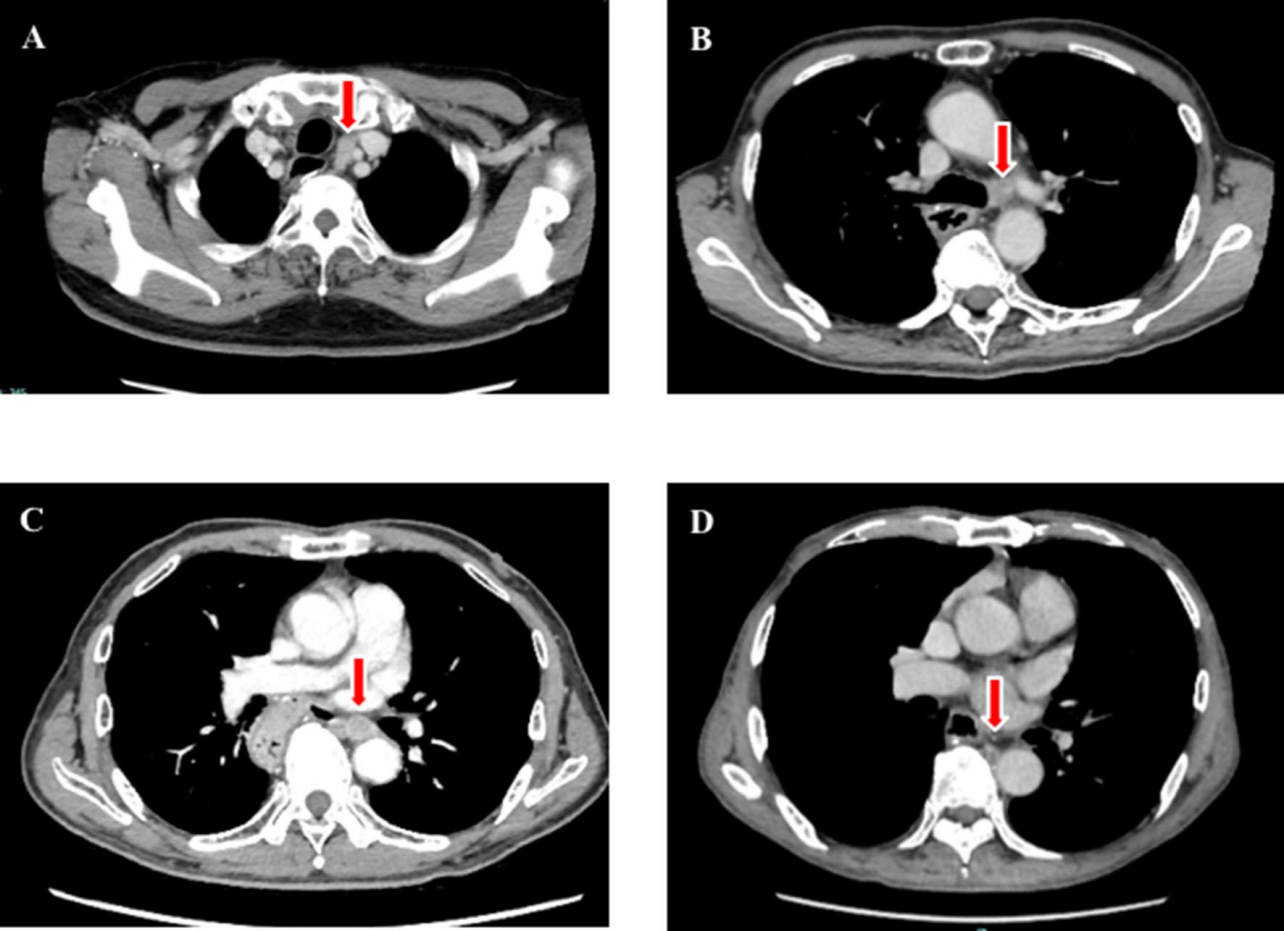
Contrast-enhanced computed tomography images of recurrent lymph nodes: (a) left recurrent laryngeal nerve lymph node; (b) left tracheobronchial lymph node; (c) left main bronchus lymph node; (d) thoracic paraaortic lymph node.
